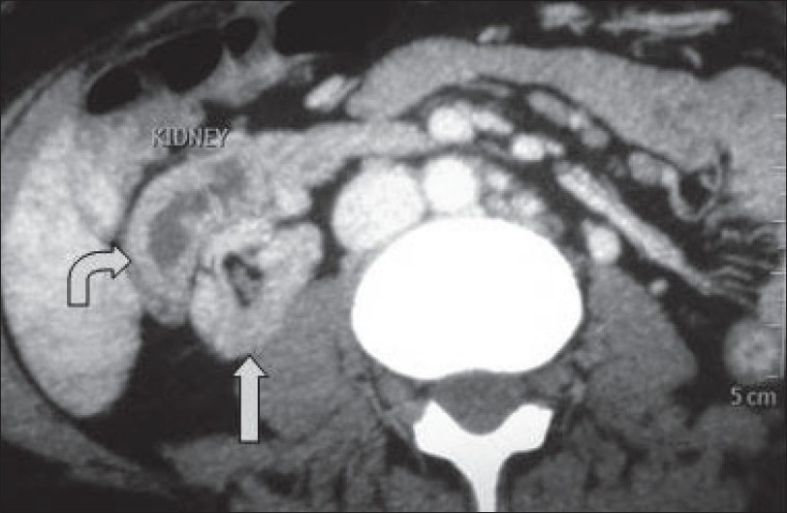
A computed tomography showing crossed fused ectopia. The ectopic kidney is situated anterolateral to the orthotopic kidney
